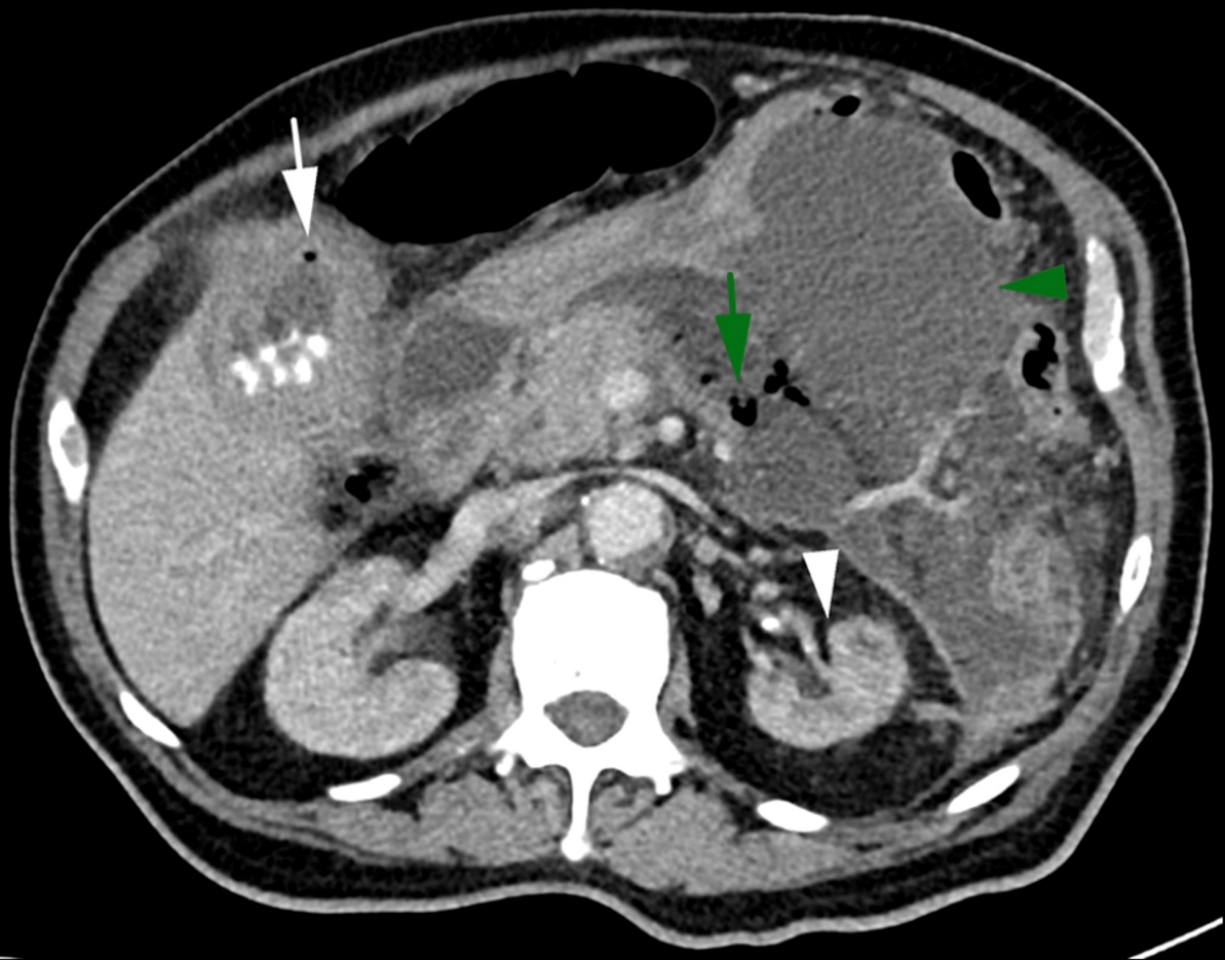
Axial contrast enhanced CT of the abdomen in the portal venous phase performed five days later demonstrating a reduction in the volume of gas within the gallbladder (white arrow), a reduction in the gas within the left kidney (white arrowhead), unchanged pancreatic gas (green arrow), and a larger, more defined peripancreatic collection (green arrowhead).
